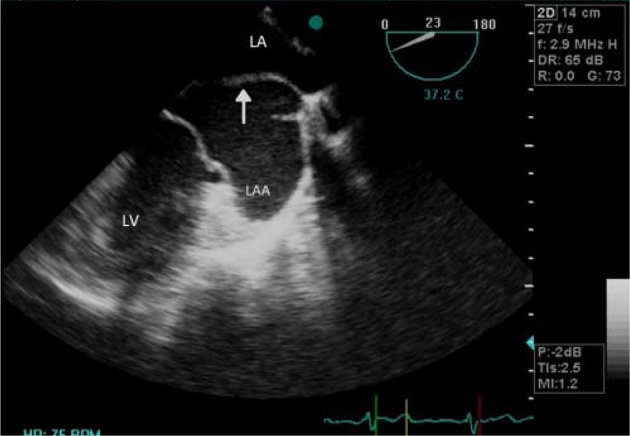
Transesophageal Echocardiography Showing Membrane Within Left Atrium (Arrow), Just Above Left Atrial Appendage LA, left atrium; LAA, left atrial appendage; LV, left ventricle.
